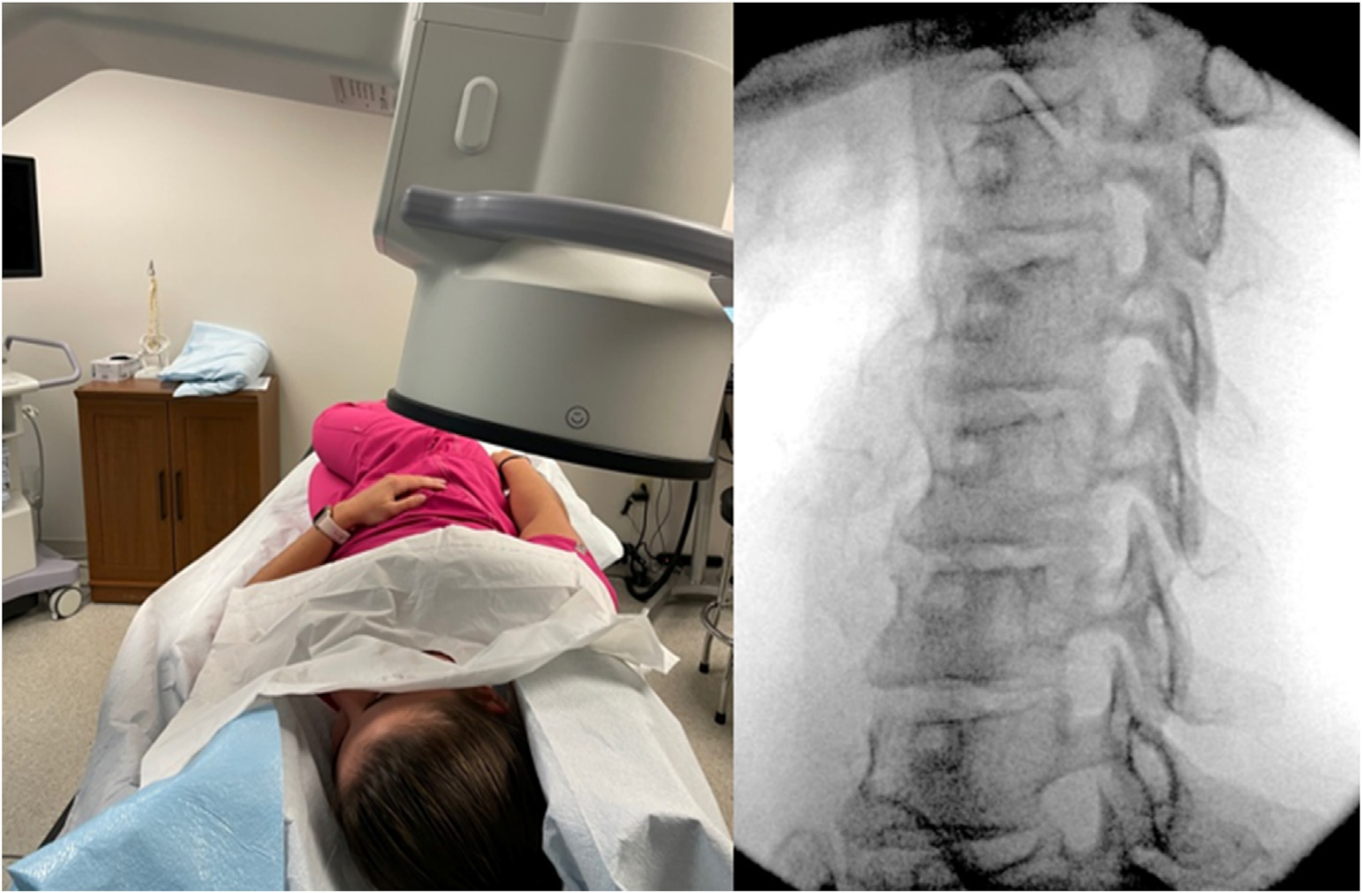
From the patients true AP position in Fig. 6, the C-arm is rotated ipsilaterally (past zero) to an angle based upon the relative angle of the patient's true AP as determined in Fig. 6. The true AP angle measurement (on fluoroscopy) is subtracted from the measured SAP angle on MRI. This follows the formula: Modified approach angle of obliquity for needle insertion ​= ​Angle of SAP measured on MRI minus the angle of the patient's true AP on fluoroscopy. In this case, the angle of the AP (Fig. 6) was determined to be 44°. The angle of the SAP on MRI was 61°. The C-arm was rotated ipsilaterally from 44°, past fluoroscopic zero degrees, to 17° (61–44 ​= ​17).
